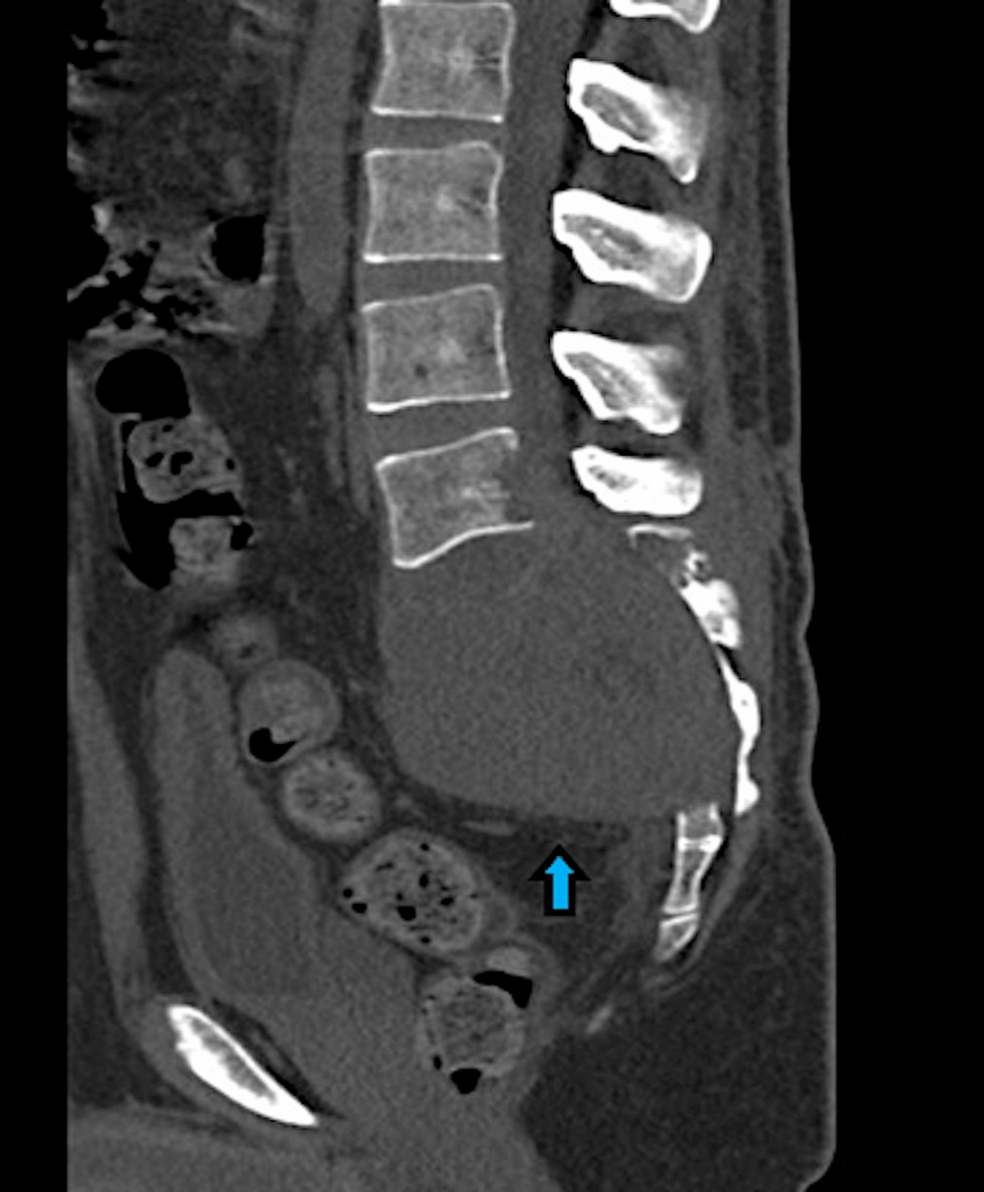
CT imaging in sagittal view showing a large giant cell tumour invading the sacrum and iliac bones (blue arrow). CT: computed tomography
